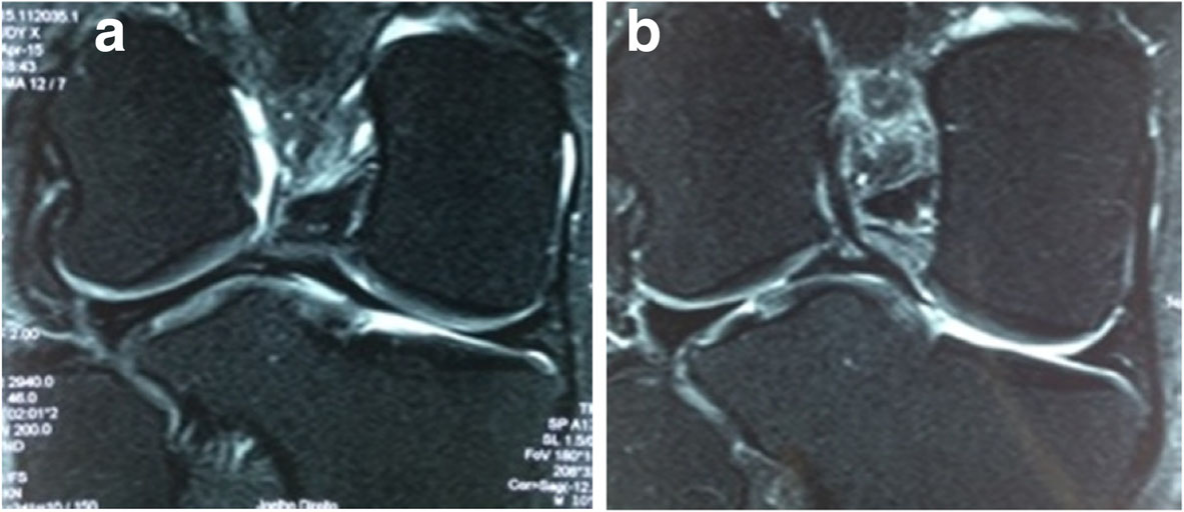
a and b Magnetic resonance imaging in coronal T2 showing the meniscal variant of posterior junction and insertion to the anterior cruciate ligament
